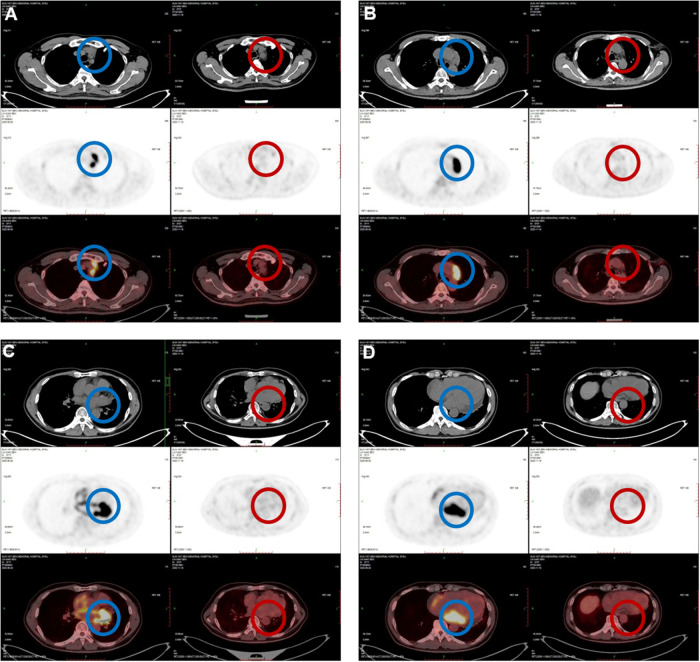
PET-CT imaging: (A) active FDG uptake of enlarged mediastinal multiple lymph nodes (SUVmax: 27.3) before treatment (in the blue circles); (B–D) active FDG uptake of the cardiac mass (SUVmax: 28.7) in the left atrium at the left AV junction (in the blue circles); (A–D) after treatment, the previous cardiac mass and enlarged mediastinal lymph nodes had disappeared completely (in the red circles).
